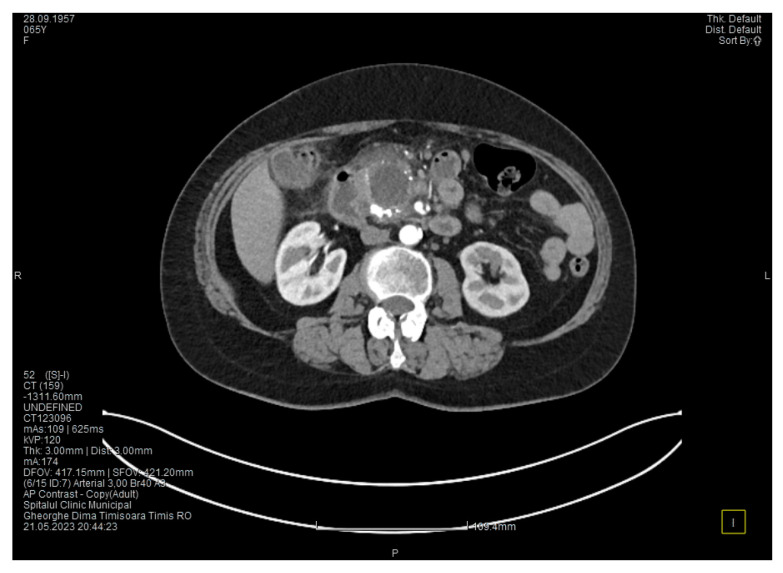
The second CT scan shows a cyst in the pancreatic head and calcifications in the pancreatic head and groove area.
